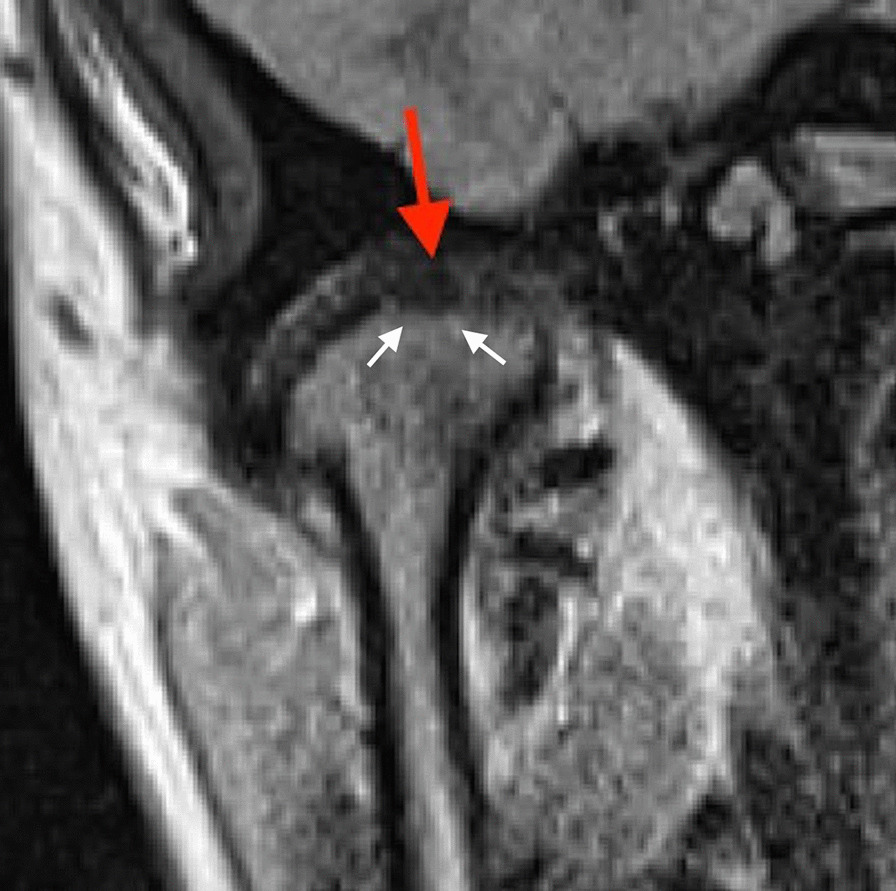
Closed–mouth-related MR images in a 18-year-old female with a 12-month- history of right TMJ pain, a TMJ pain-side-related clinical diagnosis of TMJ ‘AR and  disk displacement without reduction with limited opening (DDwoR/wLO)’, and the MRI finding of disk displacement without reduction and CE. Coronal MR image shows condyle with CE (red arrow) and bone marrow edema (white error)
